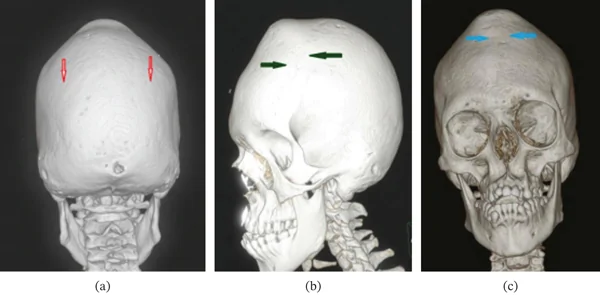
Computed tomography with three‐dimensional reconstruction of the skull showing elongated tower‐shaped skull (oxycephaly) with prematurely fused lambdoid (a, red arrows), coronal (b, green arrows), and sagittal (c, blue arrows) sutures due to craniosynostosis. All the three sutures are nonvisualized.
